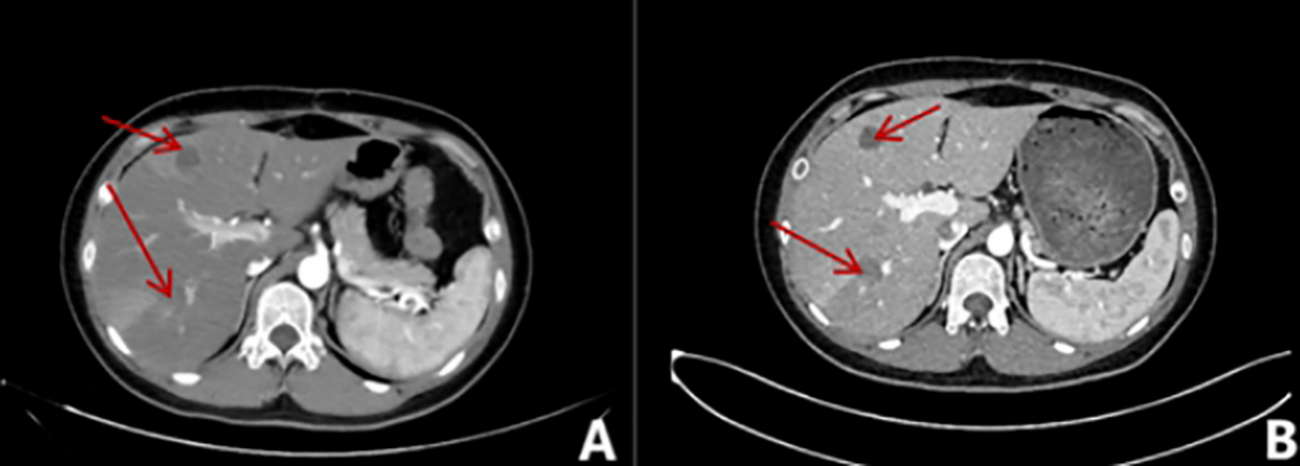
Whole abdominal contrast-enhanced CT scan. (A) A round, slightly low-density shadow is present in the right posterior lobe of the liver with unclear borders, measuring approximately 2.2x2.0cm. The edge of the shadow appears to be enhanced in the arterial phase. (B) In the right posterior lobe of the liver (IM109), a round-like slightly low-density shadow with unclear boundaries, measuring approximately 2.0cm×1.4cm, was observed. Additionally, there are multiple round-like cystic low-density shadows in the liver, ranging from 0.6cm to 2.4cm in size.
